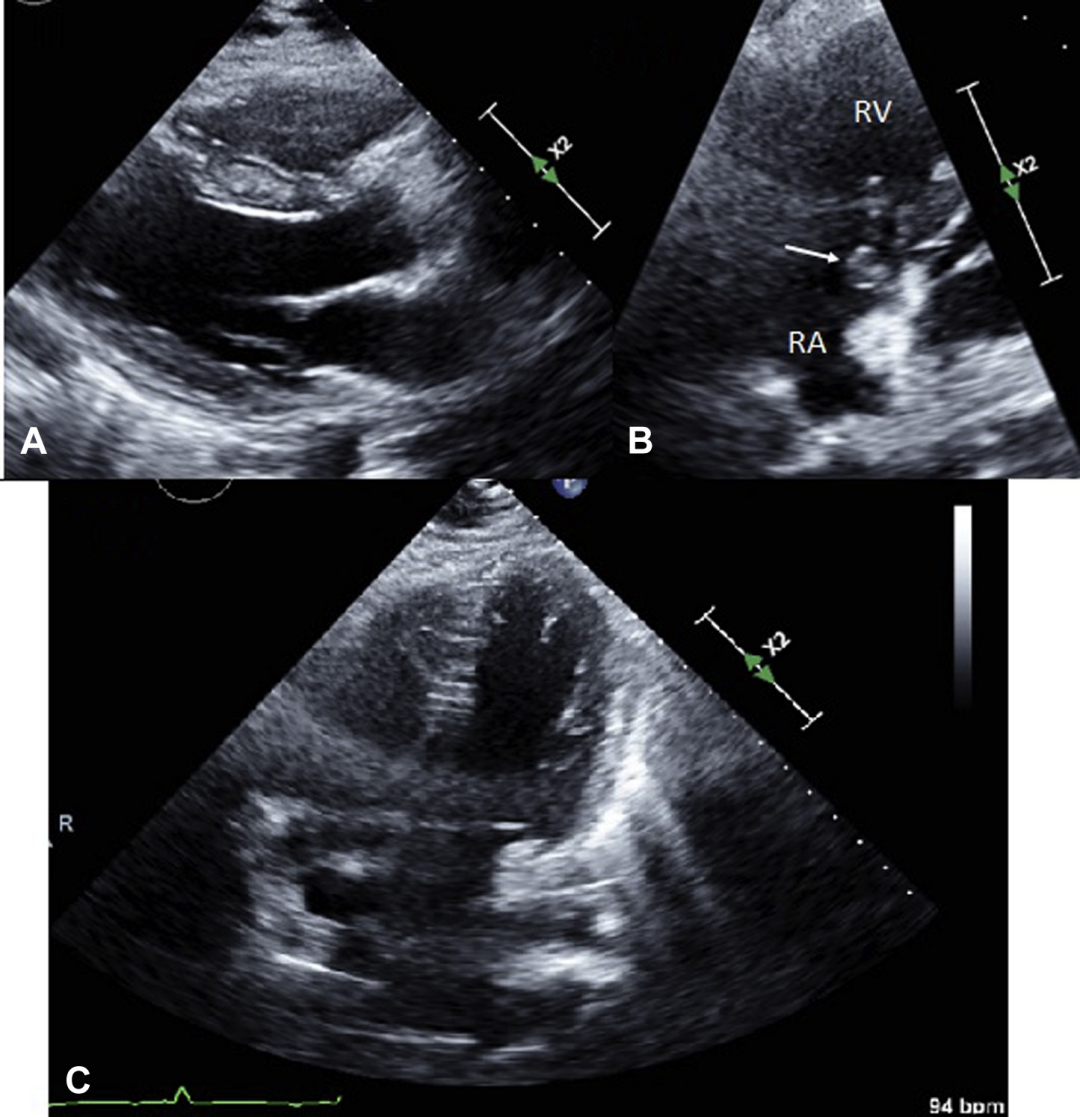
Echocardiogram upon admission. (A) Parasternal long-axis view revealed no pericardial effusion. (B) Right ventricular (RV) inflow view revealed a vegetation (white arrow) attached to tricuspid leaflet. (C) Apical 4-chamber view. RA, right atrium.
